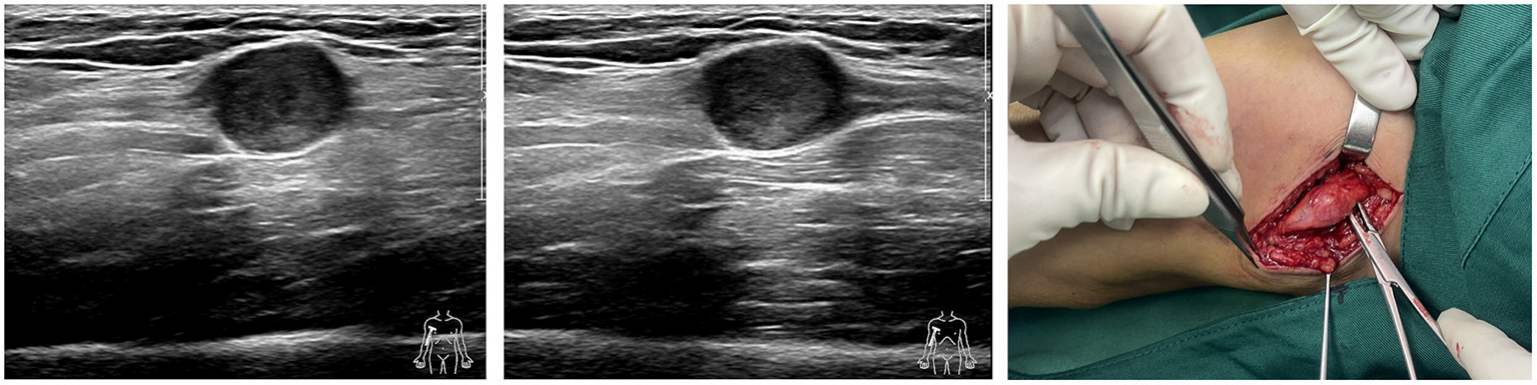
Ultrasound images and intraoperative images of schwannomas of ulnar nerve origin in the upper arm.
